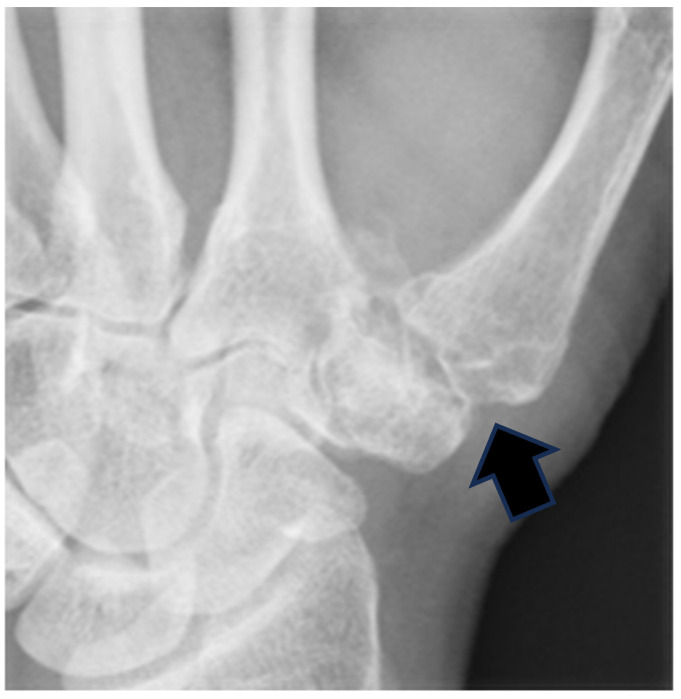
Postoperative radiograph at 2-year follow-up showing minimal proximal migration (1.2 mm) of the first metacarpal base. The black arrow indicates the preserved arthroplasty space between the base of the first metacarpal and the remaining trapezium.
